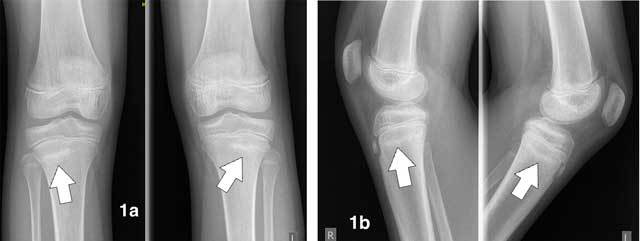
Plain radiographs of knees. a. Anteroposterior view of the right and left knee. b. lateral view of the right and left knee. Note sclerotic lines parallel to the growth plates at the lateral aspect of both proximal tibiae (white arrows) in keeping with synchronous stress fractures.
